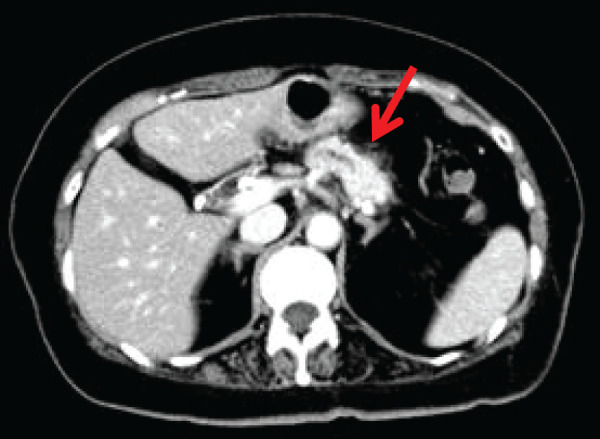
Abdominal CT (pancreatic tail). Disruption of the main pancreatic duct and mass formation were observed in the pancreatic tail.
